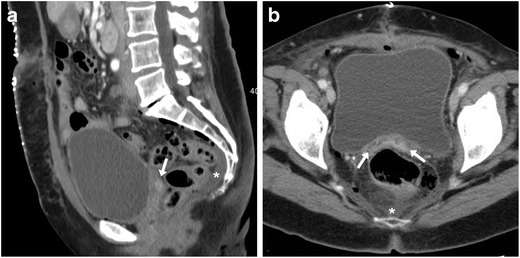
A, B) Usual contrast-enhanced CT appearance after recent abdominal hysterectomy (AH), including a non-distended vagina (arrows) with slight upwards retraction of the vaginal dome in sagittal view (A), preserved band-like transverse configuration and thin mucosal enhancement (B), minimal presacral fluid. (Partially reproduced from open access ref. no [58])
